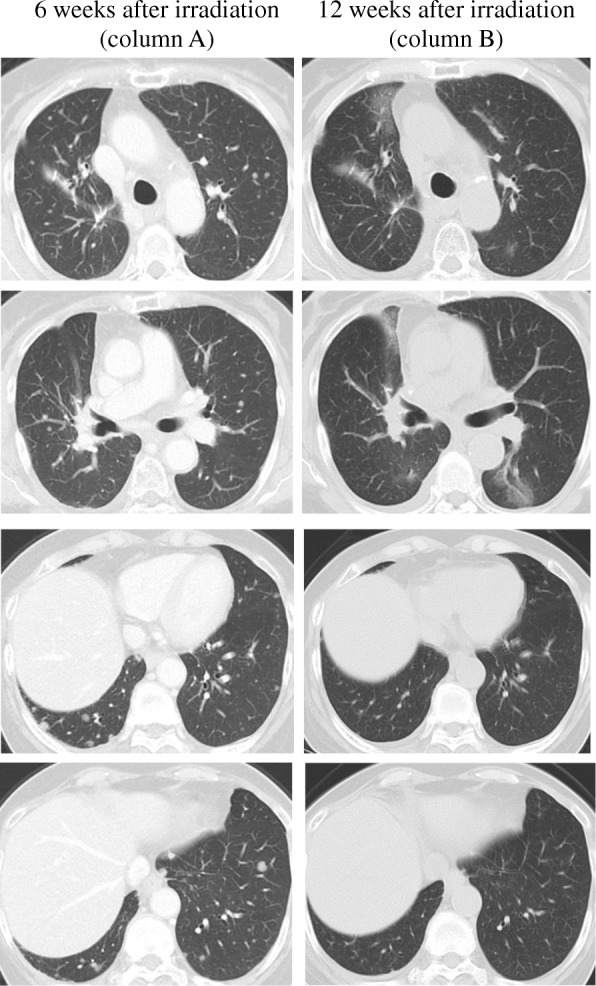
A computed tomography scan of the chest, performed 6 weeks after the irradiation therapy against multiple mediastinal and right hilar lymph node metastases, showed appearance of multiple pulmonary nodules in both lungs, suggesting metastases (column A). A follow-up computed tomography scan of the chest, performed 12 weeks after the irradiation therapy, showed complete disappearance of the multiple pulmonary metastases associated with radiation pneumonitis (column B)
